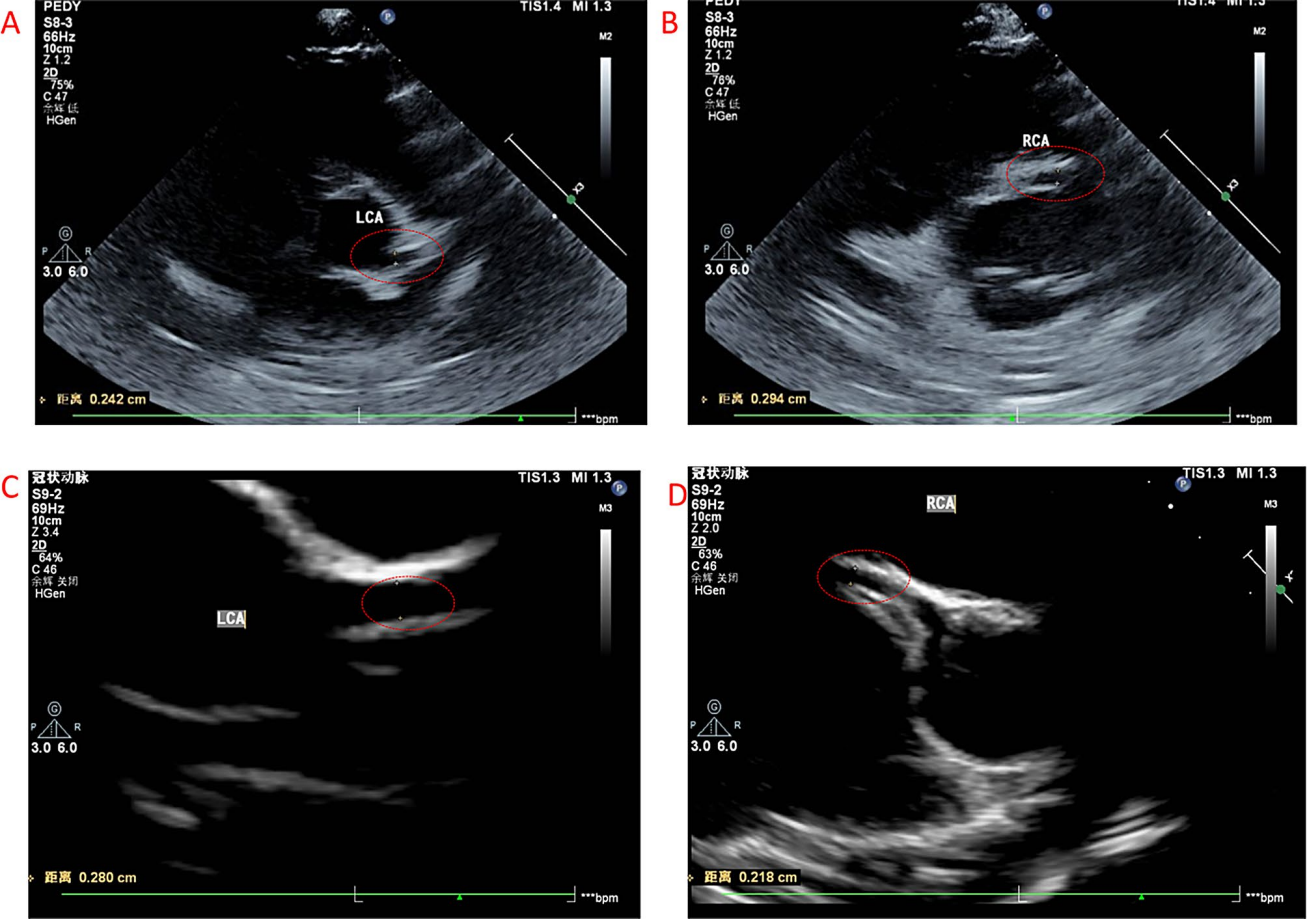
Coronary artery echocardiography before and after treatment for Kawasaki disease. A Left Coronary Artery (LCA) before treatment. B Right Coronary Artery (RCA) before treatment, showing arterial dilation. C Left Coronary Artery (LCA) after treatment. D Right Coronary Artery (RCA) after treatment, showing normalization of the vessel diameter
